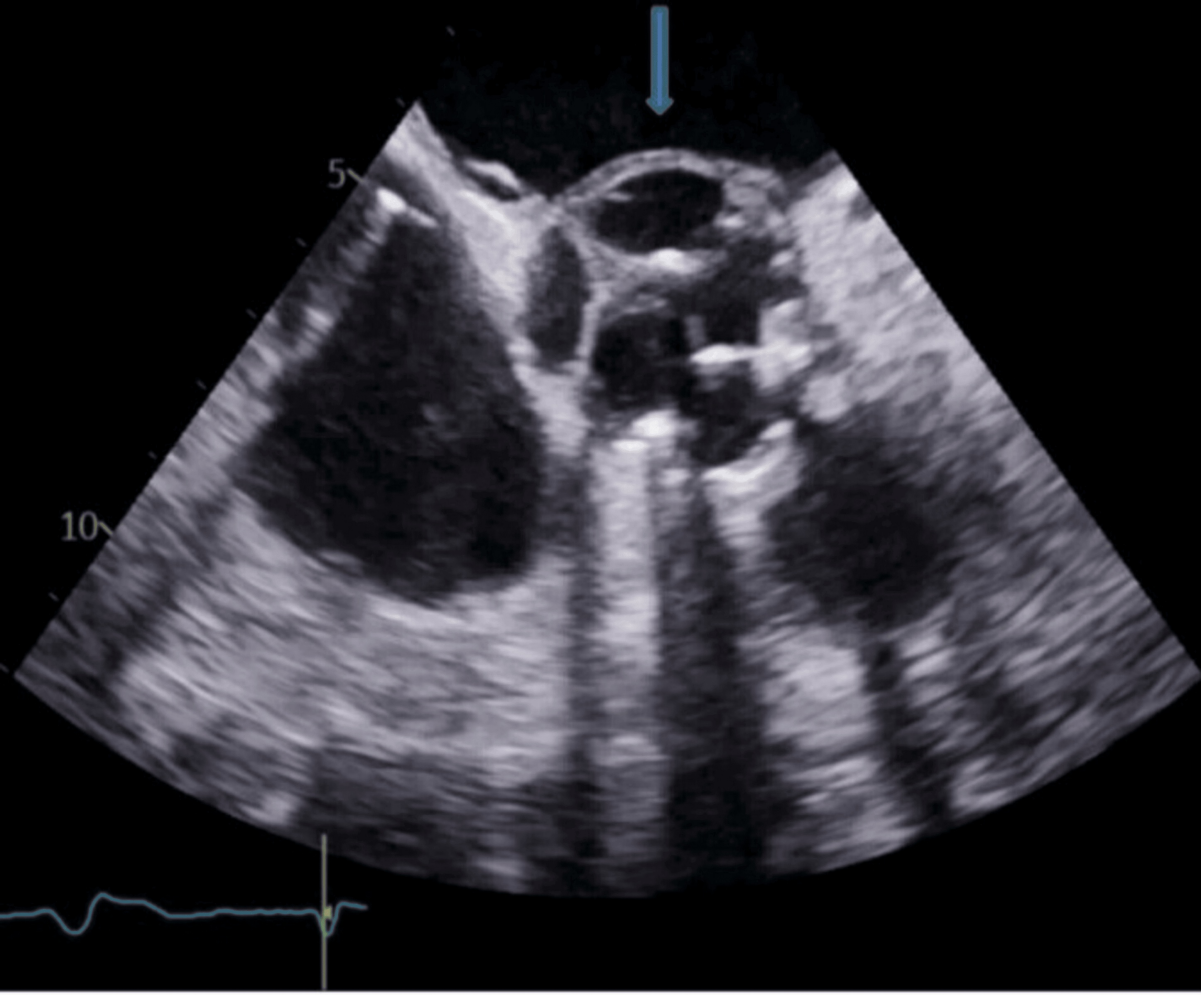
Transesophageal echocardiogram showing aortic root abscess (pointed arrow)
